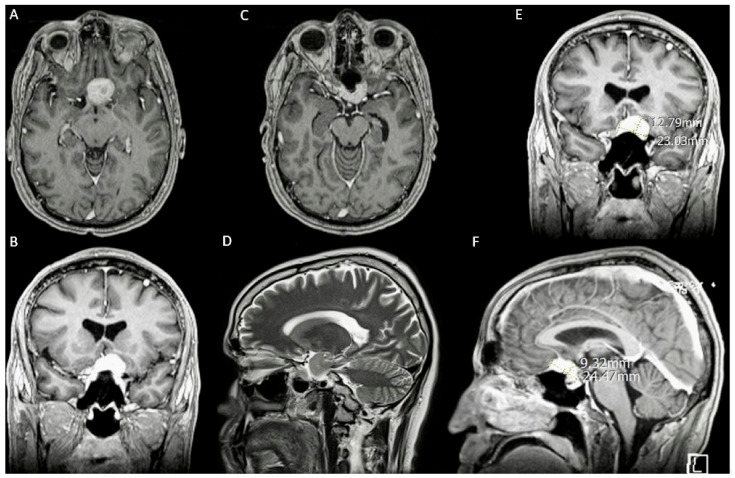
Case 1’s preoperative MRI. (A) An axial T1 view of a 1.5 cm × 2.1 cm enhancing sellar lesion. (B) A coronal T1 view of the suprasellar mass encircling the optic chiasm. (C) An axial T1 view of the mass compressing the optic chiasm and left carotid artery and encircling the left optic nerve. (D) A sagittal T2 sequence showing the close relationship of the tumor with the terminal branches of the ICA. (E) Coronal T1 view of tumor dimensions. (F) Sagittal T1 view of tumor dimensions.
